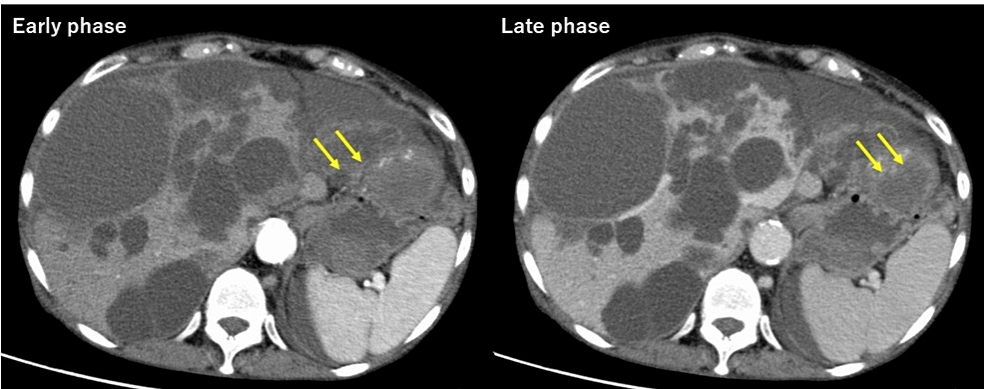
Contrast-enhanced CT scan of the abdomen on admission Contrast-enhanced CT shows multiple liver cysts. In the early contrast-enhanced phase, a strong enhancing effect is observed in cysts in the lateral zone of the left lobe of the liver. In this area in the late contrast phase, the enhancing effect is diffused, suggesting intracystic hemorrhage.
